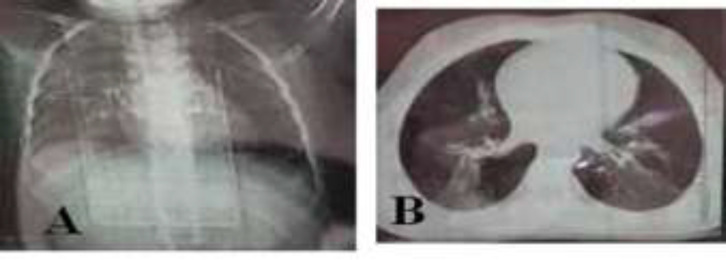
A, B CXR and lung CT findings: ground-glass opacity in both lungs
